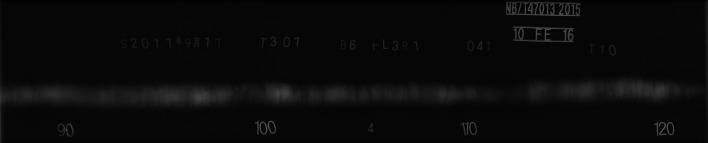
The scanned X-ray imaging film
